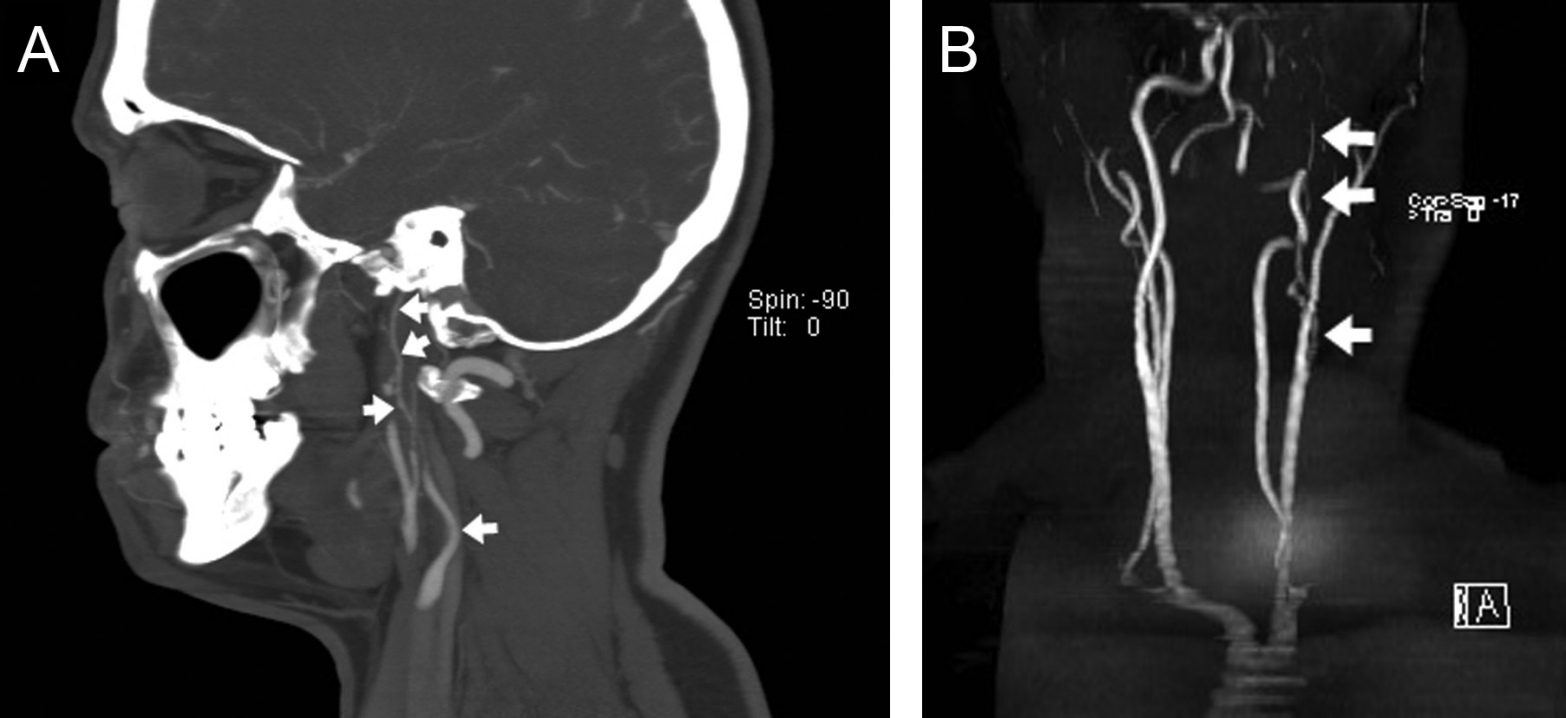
The long segment of stenosis, but not occlusion, of the left internal carotid artery (short arrows) is more definitively demonstrated on CT angiography (A) on a sagittal maximum intensity projection than on MR angiography (B) on coronal maximum intensity projection.
